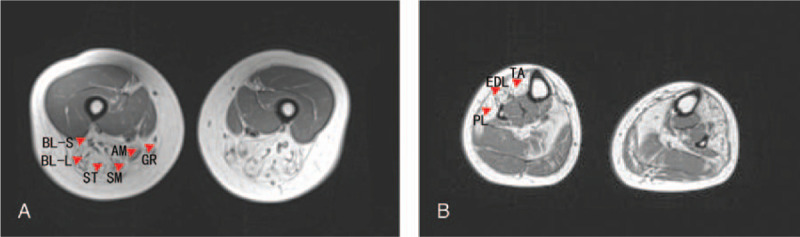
Axial-T1-weighted magnetic resonance images of the muscles showing a typical pattern of muscle involvement. The hyperintense areas reflect fatty infiltration. (A) In the thigh, the long and short head of the biceps femoris (BL-L, BL-S), semimembranosus (SM), semitendinosus (ST), adductor magnus (AM), and gracilis (GR) show moderate fatty infiltration. (B) In the lower leg, the tibialis anterior (TA), extensor digitorum longus (EDL), peroneus longus (PL) show conspicuous fatty changes (red arrows).
